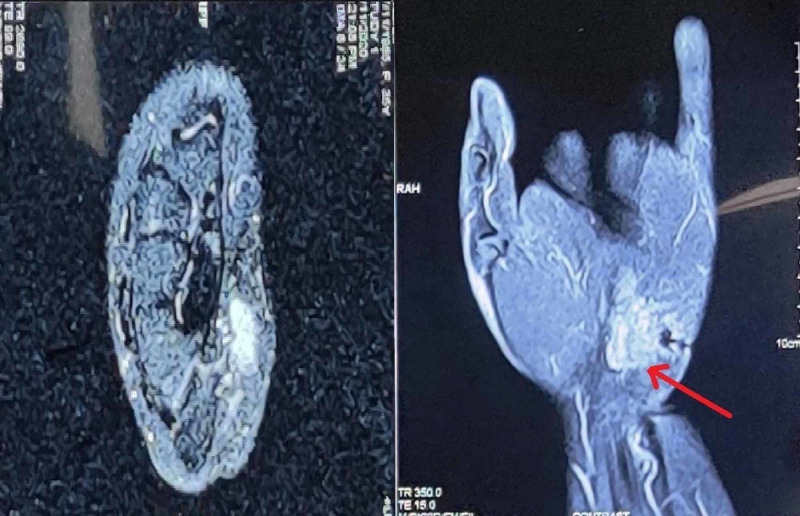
MRI left hand showing a well-defined cystic lesion with internal loculations MRI: magnetic resonance imaging
